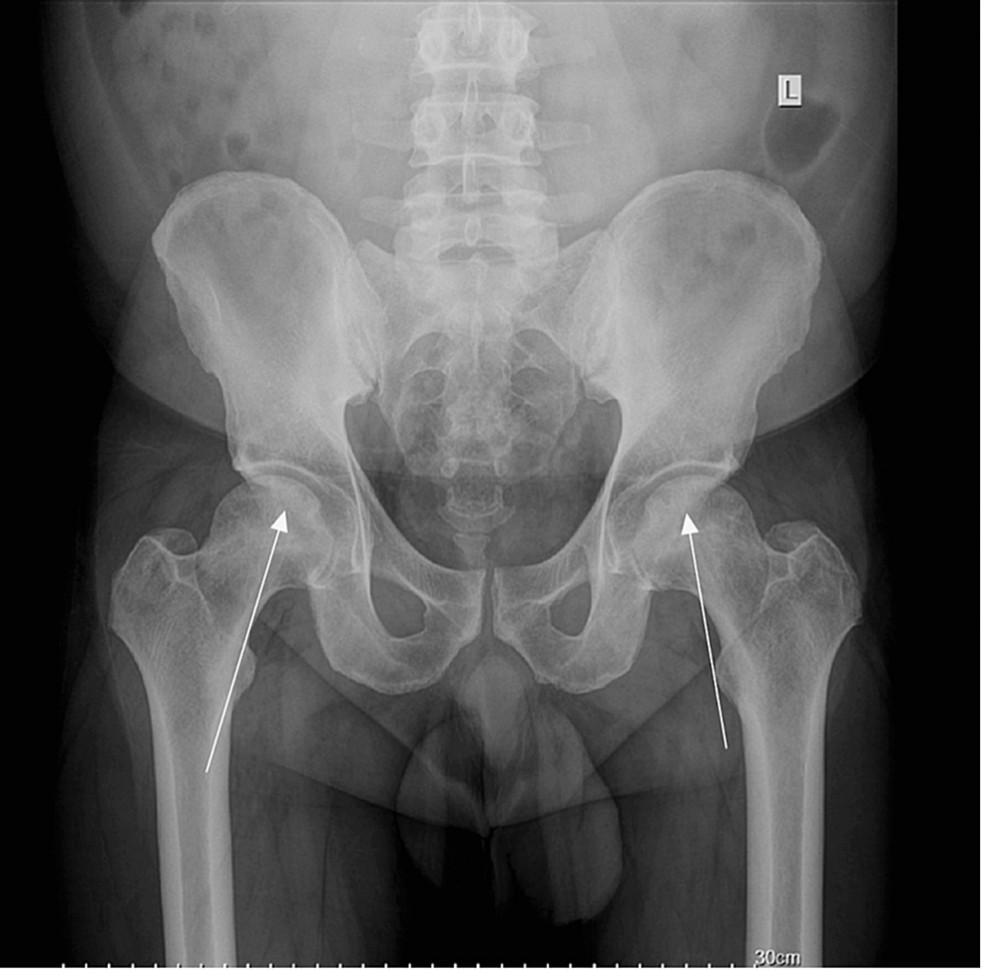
Anteroposterior radiograph of the pelvis. Arrows indicate stage II bilateral femoral head and left distal femur avascular necrosis without collapse.
